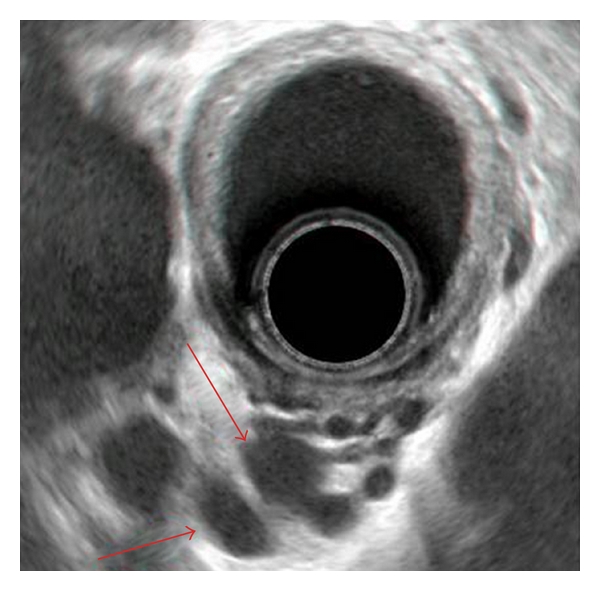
“Deep” oesophageal varices (red arrows) on endosonographic examination.
